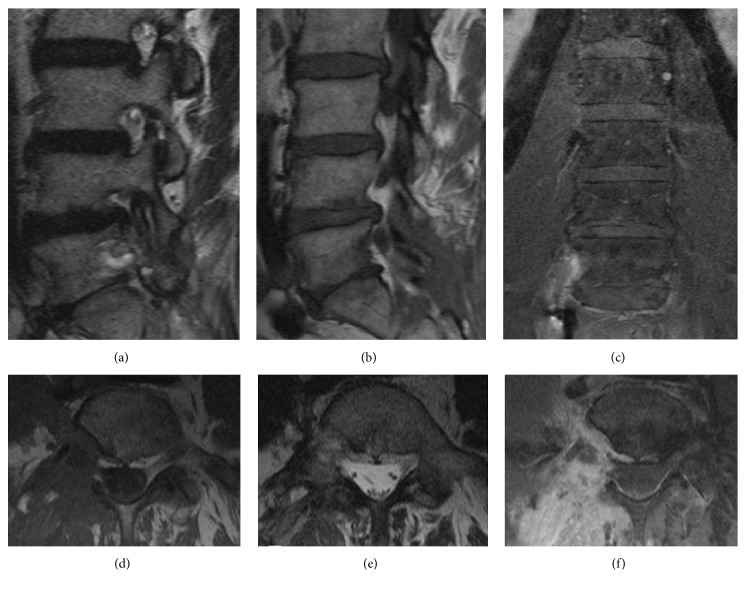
Postoperative spinal MRI. The mass was removed from the vertebral foramen up to the psoas muscle (a, b, and d). Resection of the lesion medially to the psoas muscle was achieved despite its firm adherence to the muscle itself and the risk of vascular injury (c, e, and f).
